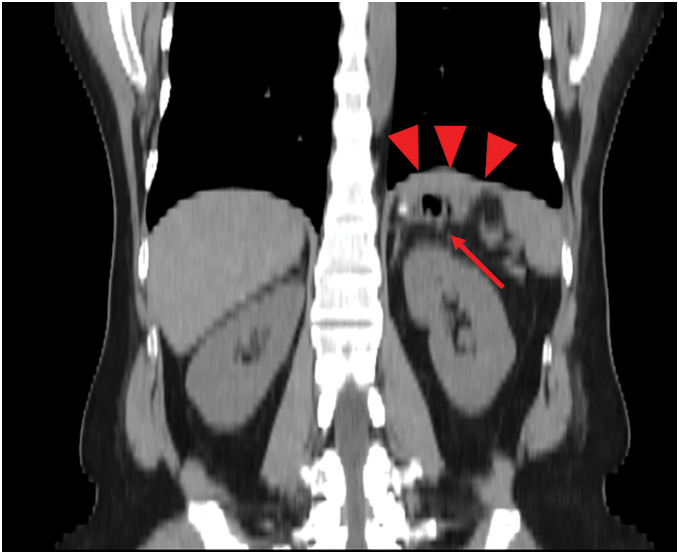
Representative image of postoperative CT. Postoperative CT image at the 1-month follow-up. The arrowheads show the diaphragm; the arrow shows the stomach. No recurrence of the parahiatal hernia was seen.
